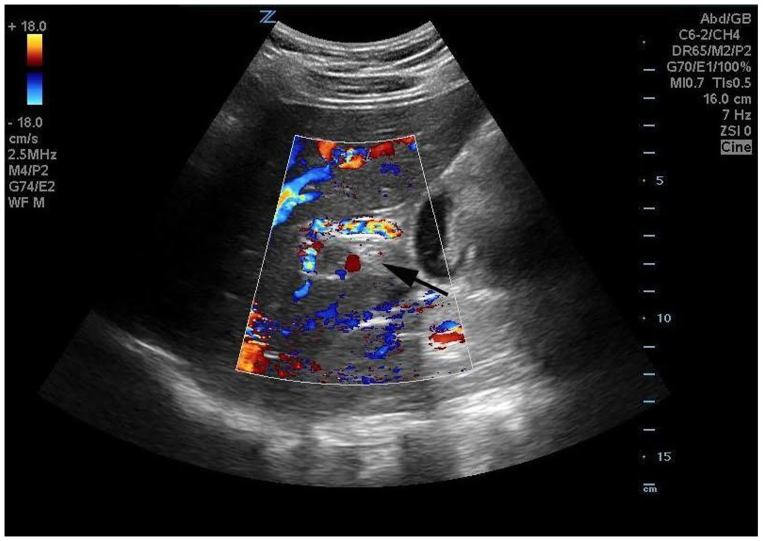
Subcostal oblique parasagittal image with color flow Doppler demonstrates a lack of color flow in the portal vein (black arrow).
